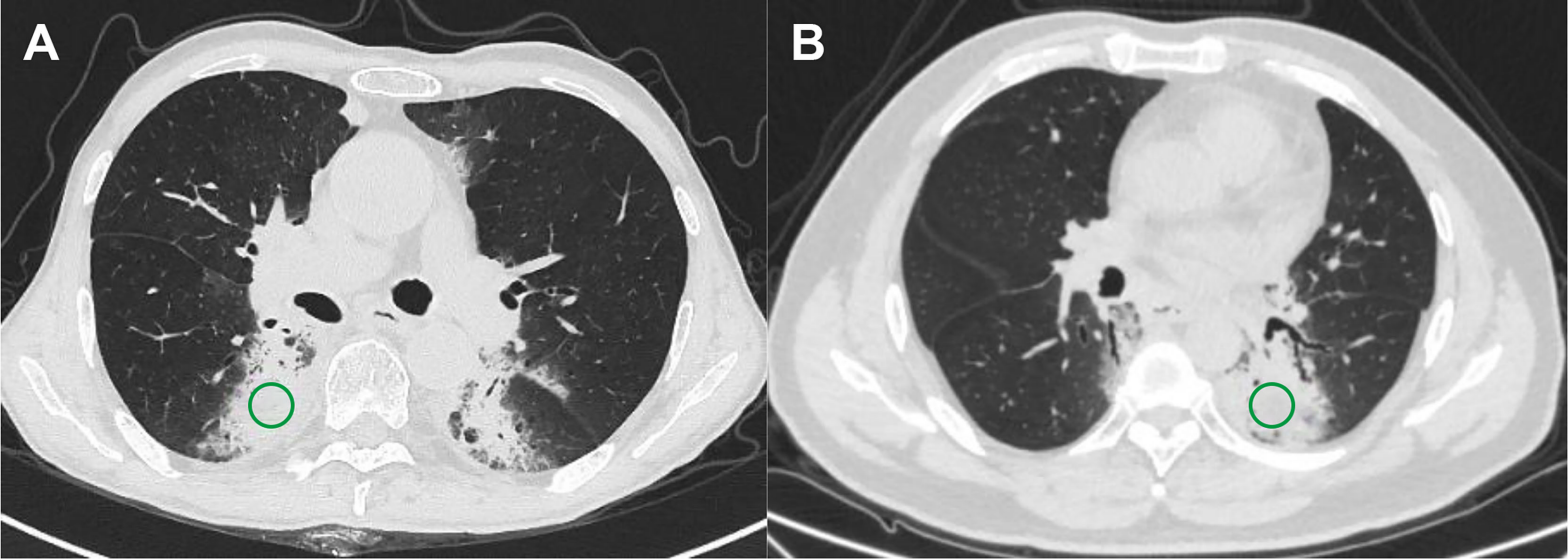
Examples of regions of interest for two subtypes of pneumonitis. (A) Checkpoint inhibitor-related pneumonitis on routine CT and (B) radiation pneumonitis on routine CT.
